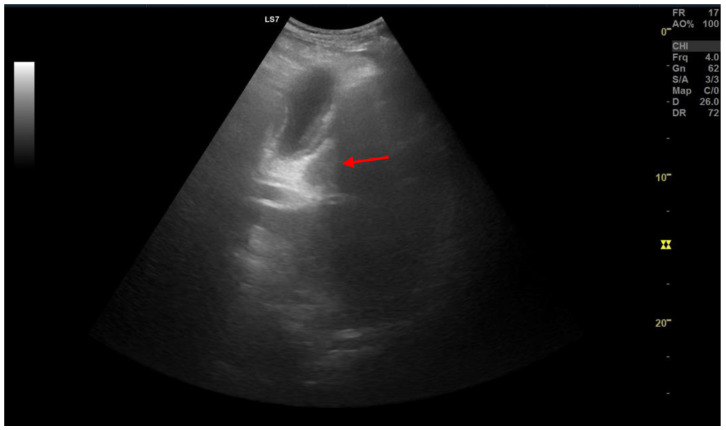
Ultrasonography of a case with type 3 pancreatic heterotopy (red arrow).
